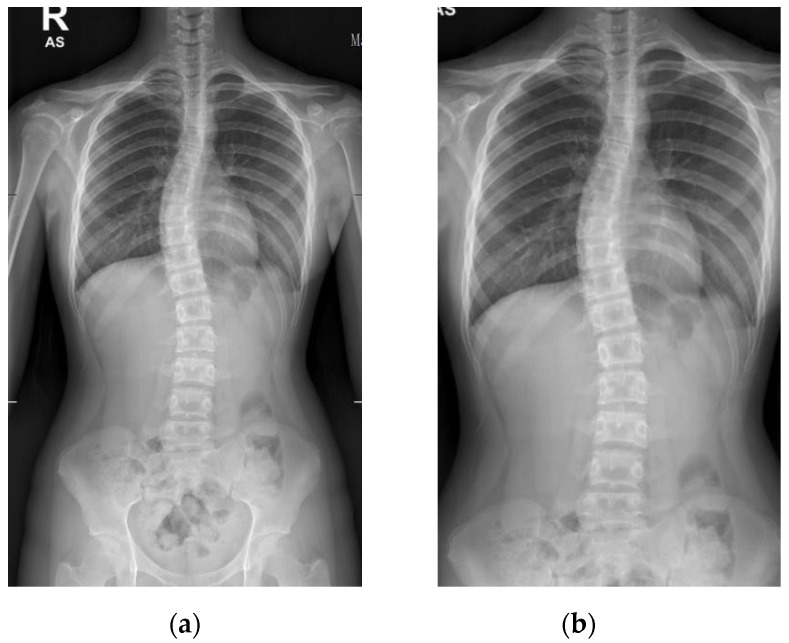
Pre-processing of the X-ray image: (a) original; (b) post-processing.
